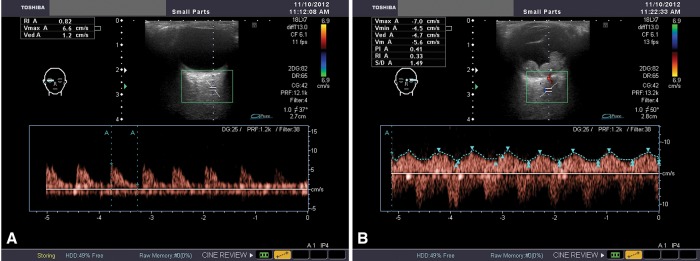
(A) Color Doppler image of the right eye, which was the contralateral normal eye, showing a reduced peak velocity in the central retinal artery and central retinal vein compared with that in tumor-containing eyes. (B) Color Doppler image of the left eye, which was the tumor-containing eye of the same patient as shown in (A); the velocities (min., max. and average), resistivity index and pulse index of the central retinal vein are displayed in the upper left-hand square. The wave boxes were inverted to allow automatic calculations for the vein. The blue ‘A’ and cross were added manually.
